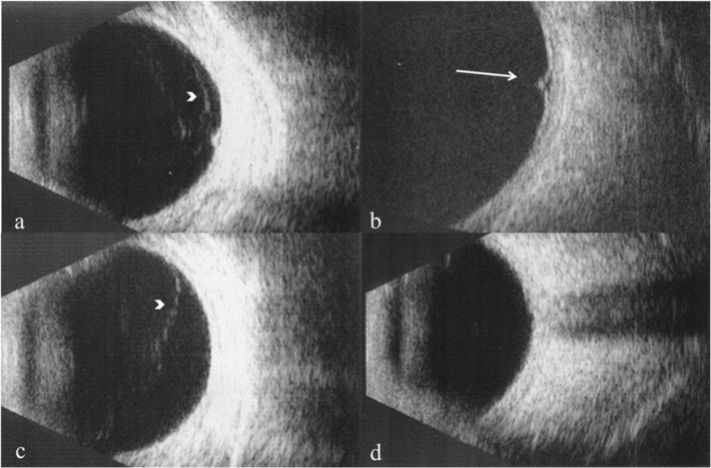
B-scan ultrasonography showing extent of vitreous detachment. Retinal tear with vitreo-retinal traction (a); retinal tear (arrow) (b); anterior vitreous detachment (arrowhead) (c); and posterior vitreous attachment (d)
